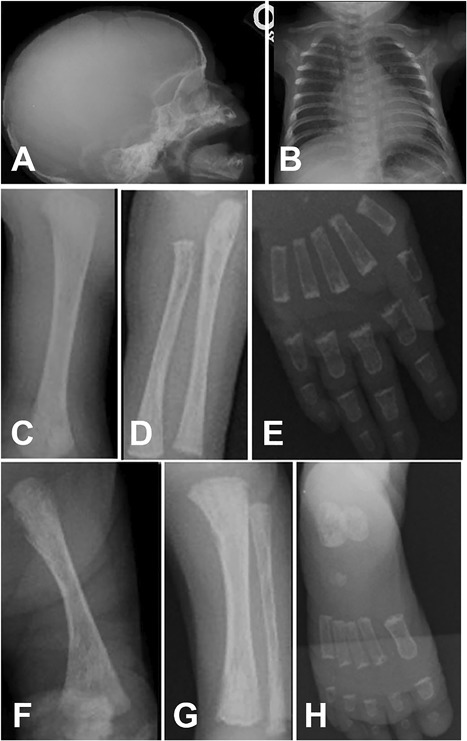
The radiographic survey on admission failed to show fibrous dysplasia lesions. In contrast, a mild‐to‐moderate degree of osteopenia was observed in diverse skeletal segments. A = cranium; B = chest; C = humerus; D = radius and ulna; E = carpal and metacarpal bones; F = femur; G = tibia and fibula; H = tarsal and metatarsal bones.
